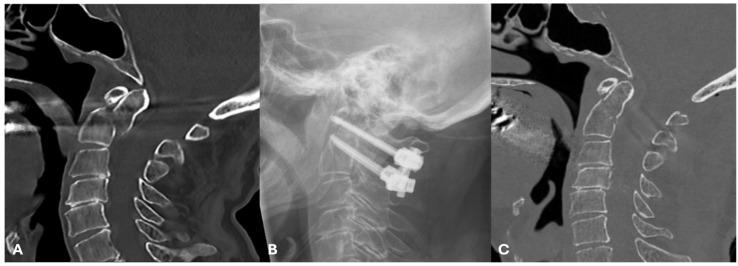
Clinical Case of an Anderson and D’Alonzo type II, Roy-Camille type II odontoid fracture: (A) Sagittal CT showing the OF, with some minor posterior displacement of C1 relative to C2, (B) postoperative lateral radiograph, showing a posterior C1–C2 fixation using the Harms-Goel technique, and (C) postoperative midline sagittal CT of the cervical spine showing good realignment of the odontoid post fixation.
